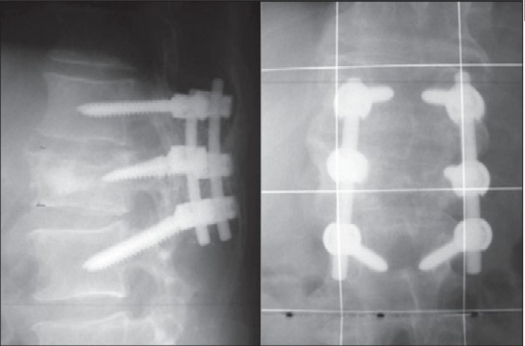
X-ray lumbar spine (Lateral and AP) of the same patient three months postoperatively showing excellent alignment following short fixation
